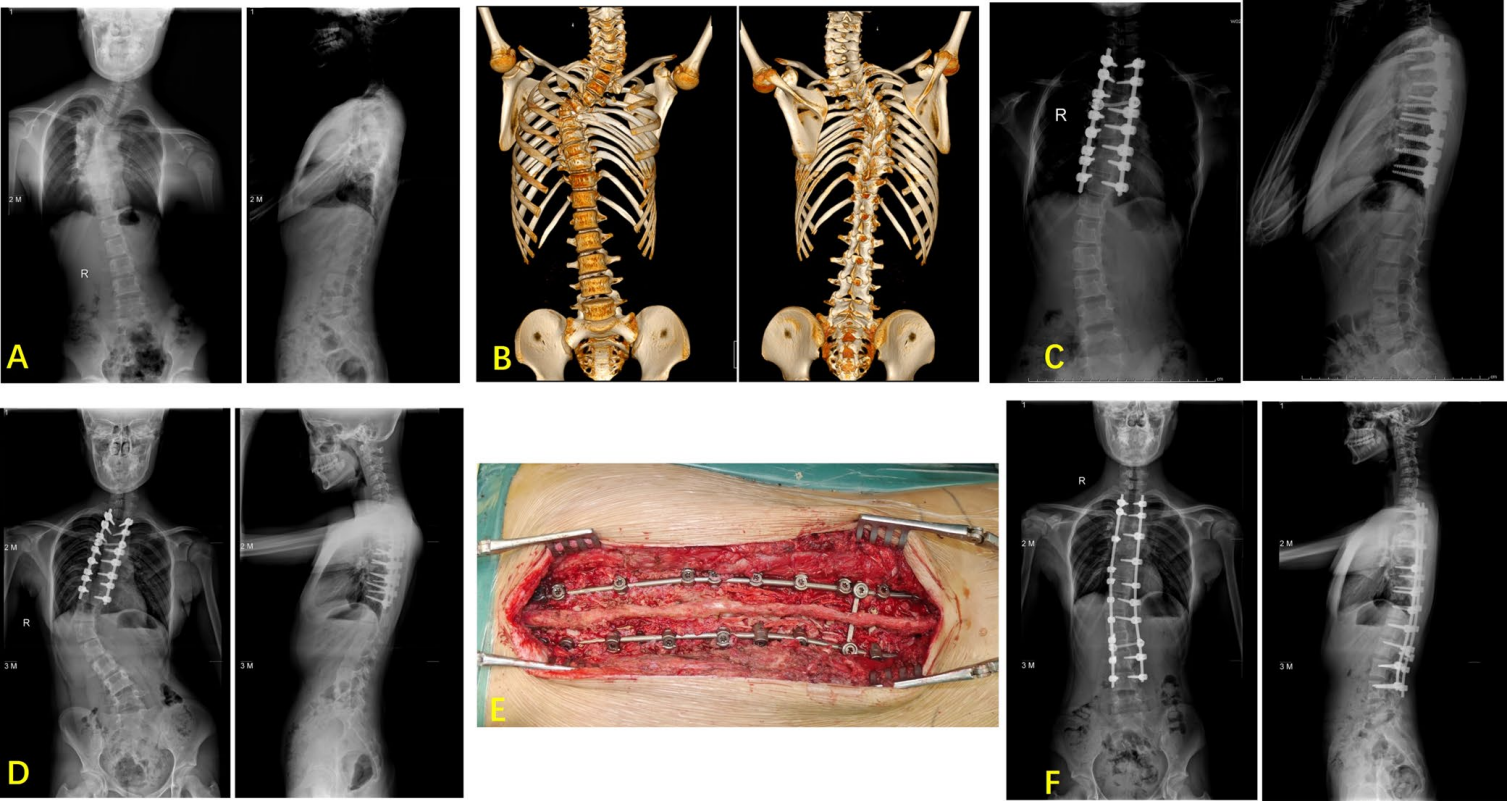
A 10‐year‐old female with congenital scoliosis. (A) Preoperative long‐standing scoliosis radiographs. (B) Preoperative three‐dimensional computed tomography scans showed congenital hemivertebra at T5 and T6. (C) Single‐stage posterior hemivertebra resection of T5 and T6, and bilateral pedicle screw fixation from T2 to T11 was performed. (D) Spinal deformity progression was found during the follow‐up. (E) Intraoperative photograph showed an unplanned revision surgery that was performed during intermediate term (2.4 years postoperatively). (F) The full‐spine radiographs after unplanned revision, with a distal fusion level to L4.
